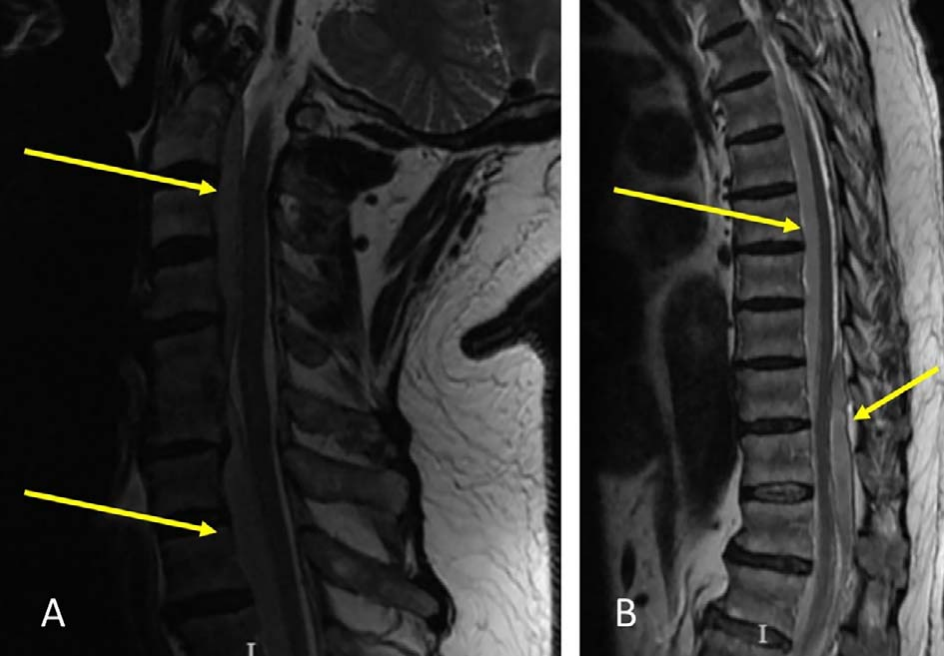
Preoperative sagittal T2 magnetic resonance imaging sequence of (A) the cervical spine and (B) the thoracolumbar spine shows epidural abscess formation (arrows).
